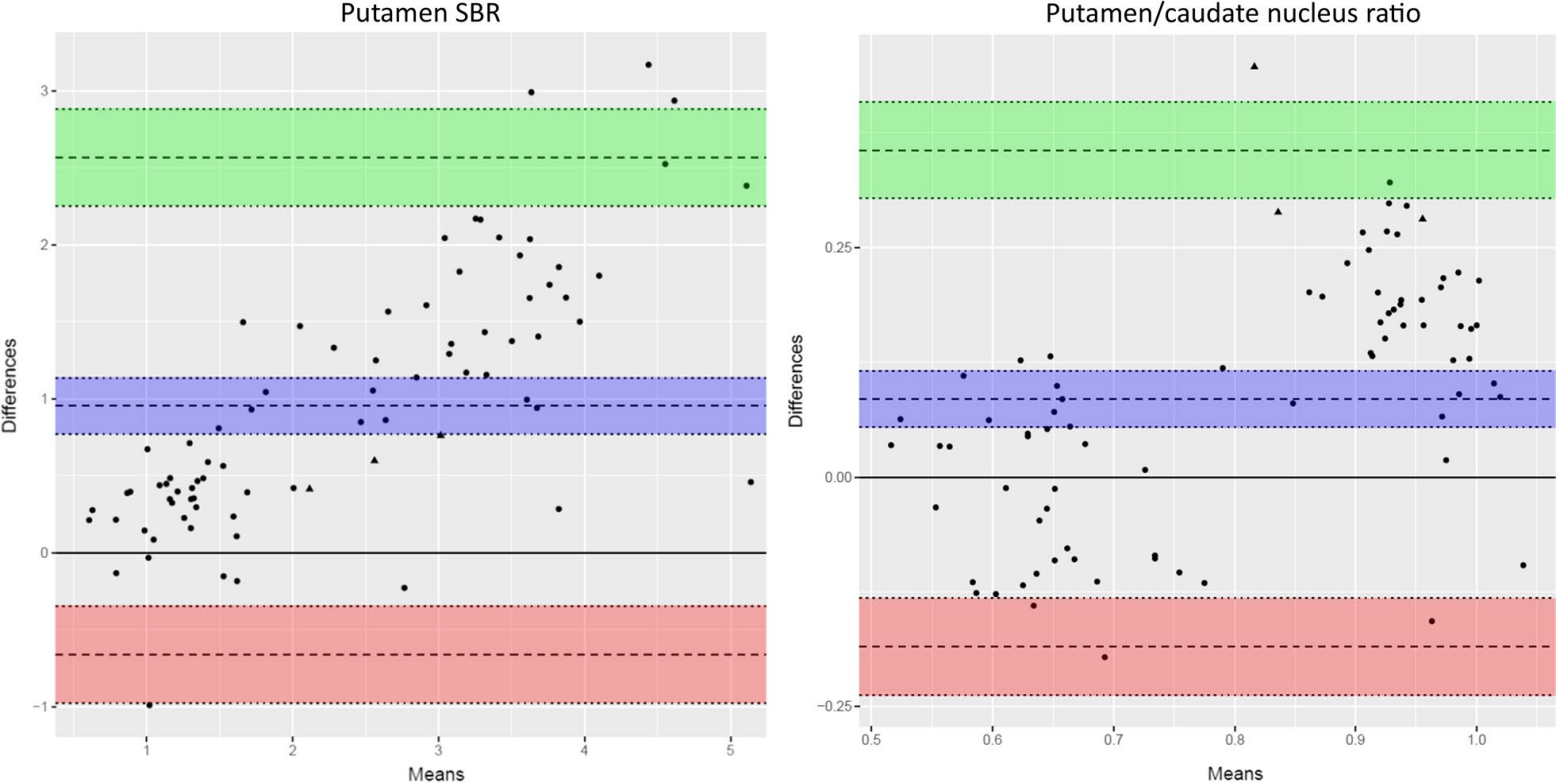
PET versus SPECT Bland–Altman plots. Same data as in Fig. 5 shown as Bland–Altman plots. Triangles represent patients with discrepancy between PET and SPECT reading. The purple area represent the mean difference with 95% confidence limits (C.I.), the green area represent the upper limit of agreement with 95% C.I., and the red area the lower limit of agreement. As expected PET show relative higher values for putamen SBR (left) for PET compared to SPECT with 95% of patients being within 50% difference. Putamen/caudate nucleus ratio (right) show similarly relatively higher values but the difference is smaller and within 35%
